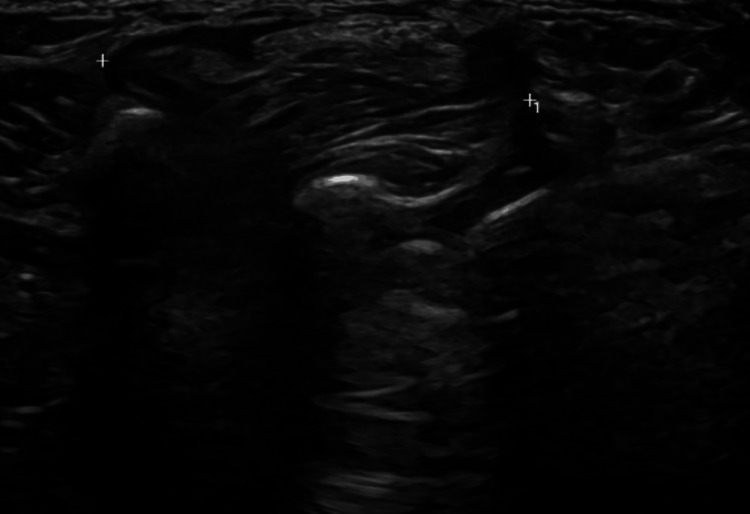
Ultrasound image of the left dorsal foot region showing a 2.8 cm x 4.8 cm x 1.1 cm soft tissue mass, located superficially to the tarsal bones with posterior extension toward the intertarsal joints. The mass is well-encapsulated, exhibiting altered echotexture with multiple echogenic stripes, but no internal vascularity, calcification, or cystic features.
